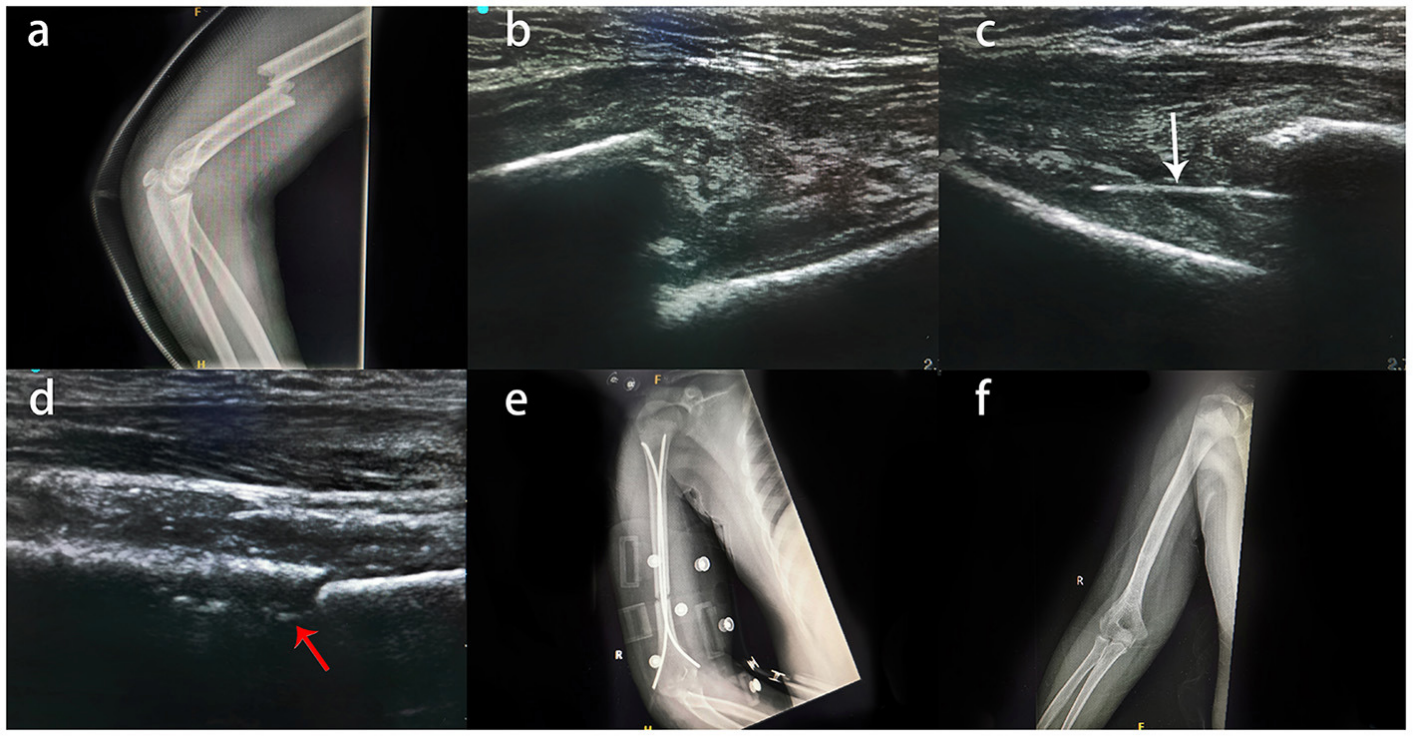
Typical case of an 11-year-old boy with right humeral shaft fracture treated by US-guided closed reduction and ESIN fixation. (A) X-ray examination of the child after injury revealed a middle humeral shaft fracture. (B) Intraoperative US showed that there was no soft tissue or nerve incarceration at the fracture site, and closed reduction was possible. (C) ESIN just past the fracture (white arrow). (D) ESIN in the bone marrow cavity after closed reduction (red arrows); it was not detectable in every patient by US. (E) Reexamination by radiography on the first day after the operation. (F) Good fracture healing was observed by X-ray at the 12-month follow-up.
